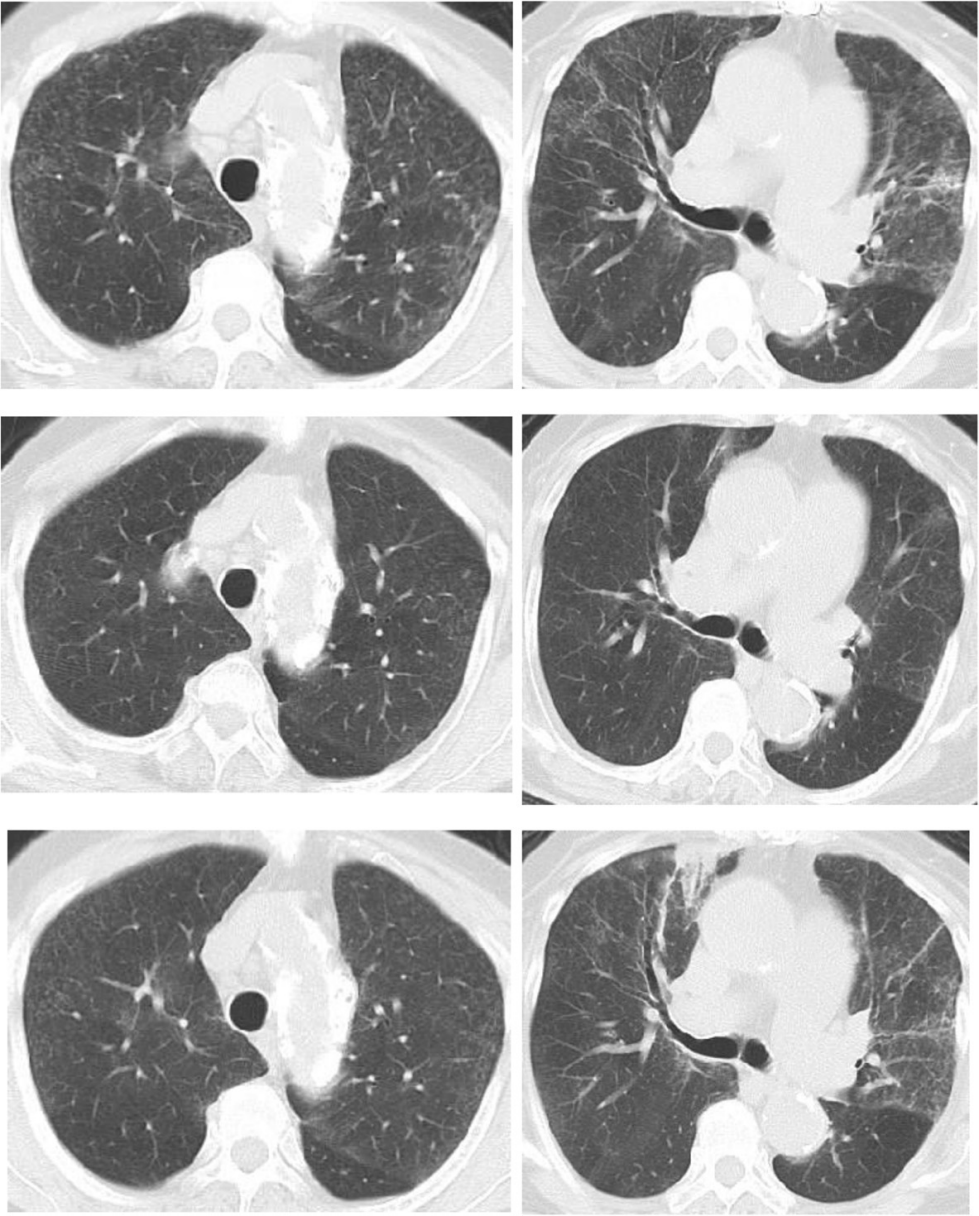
A thoracic CT before admission revealed diffuse fine centrilobular nodules with patchy ground-glass opacities of the upper lung predominance (*upper*). After the admission, the pulmonary parenchymal involvements were spontaneously improved (*middle*). 15 h after the end of the provocation test, fine centrilobular nodules and patchy ground-glass opacities appeared again. Bronchial wall thickening with peripheral airspace consolidation in the right upper lobe were also visible (*lower*)
